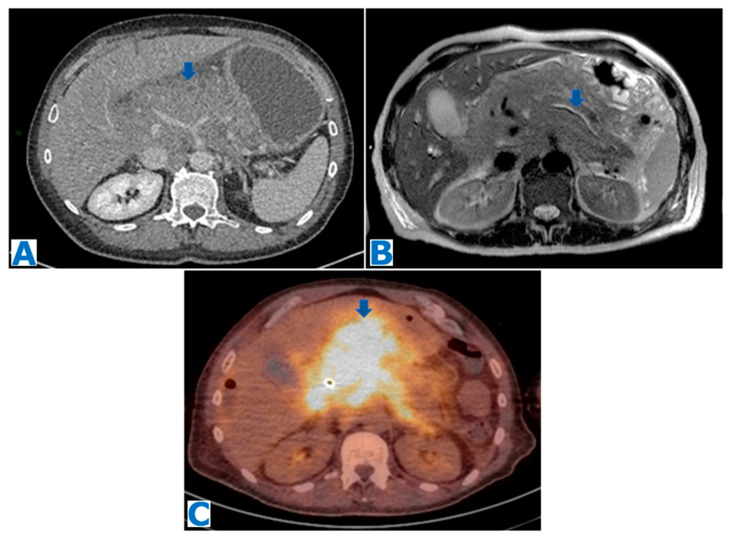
(A): axial CE portal venous phase CT, (B): axial T2WI, (C): FDG-PET-CT. Primary pancreatic lymphoma (diffuse form) in a 65-year-old alcoholic patient referred to our institution after being diagnosed with a pancreatic mass. An ill-defined infiltrating pancreatic mass is observed (arrow in (A)), with no biliary or pancreatic duct dilatation (observe the MPD’s normal appearance, arrow in (B)). The mass shows an intense hypermetabolic uptake on the FDG-PET-CT (arrow in (C)). Biopsy revealed a high-grade PPL with diffuse big cell B lymphoma and Burkitt-like components.
